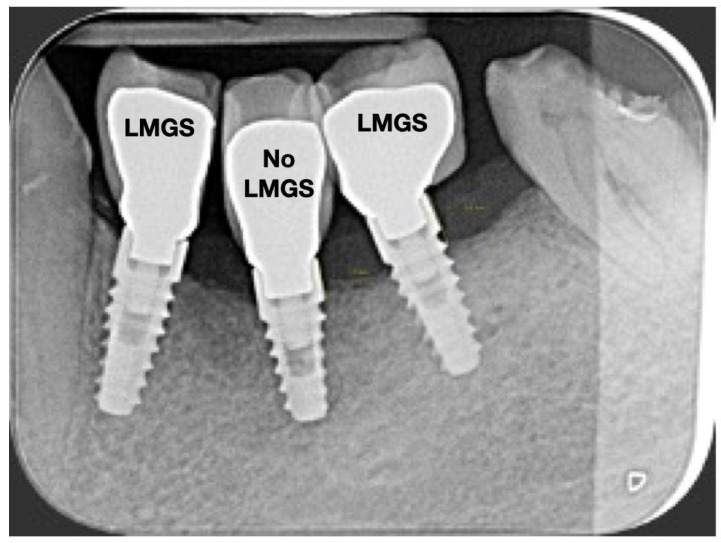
Radiograph of three (3) implants at crown delivery. (LMGS = with a laser-microgrooved collar surface; No-LMGS = without a laser-microgrooved collar surface).
